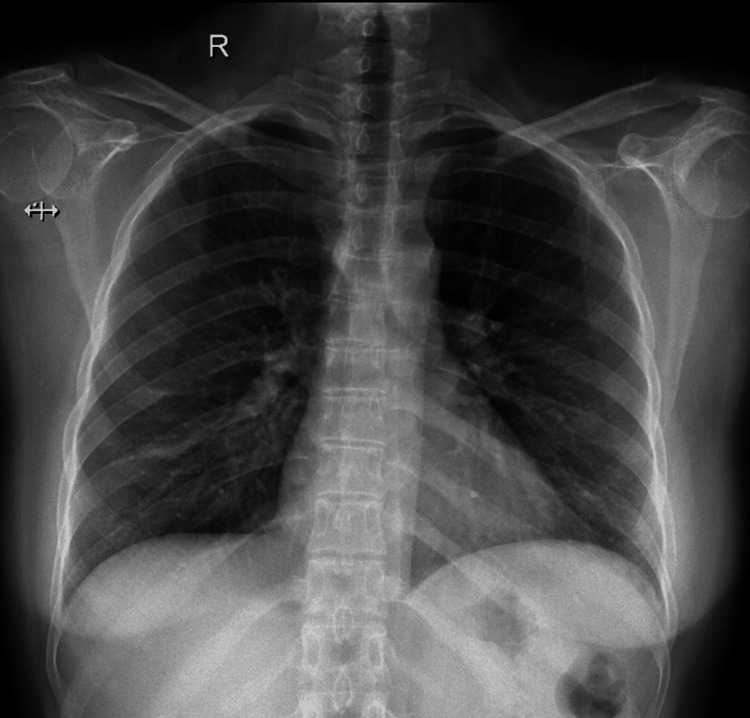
Chest X-ray showing no obvious pleuro-parenchymal abnormality.
